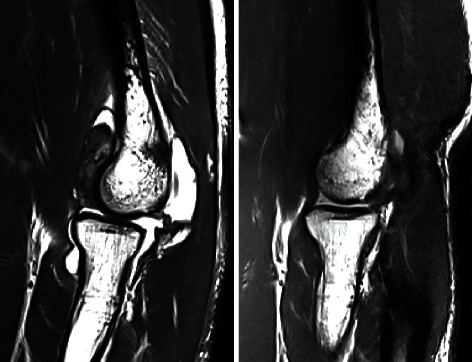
Preoperative and 6-month postoperative MRI.
